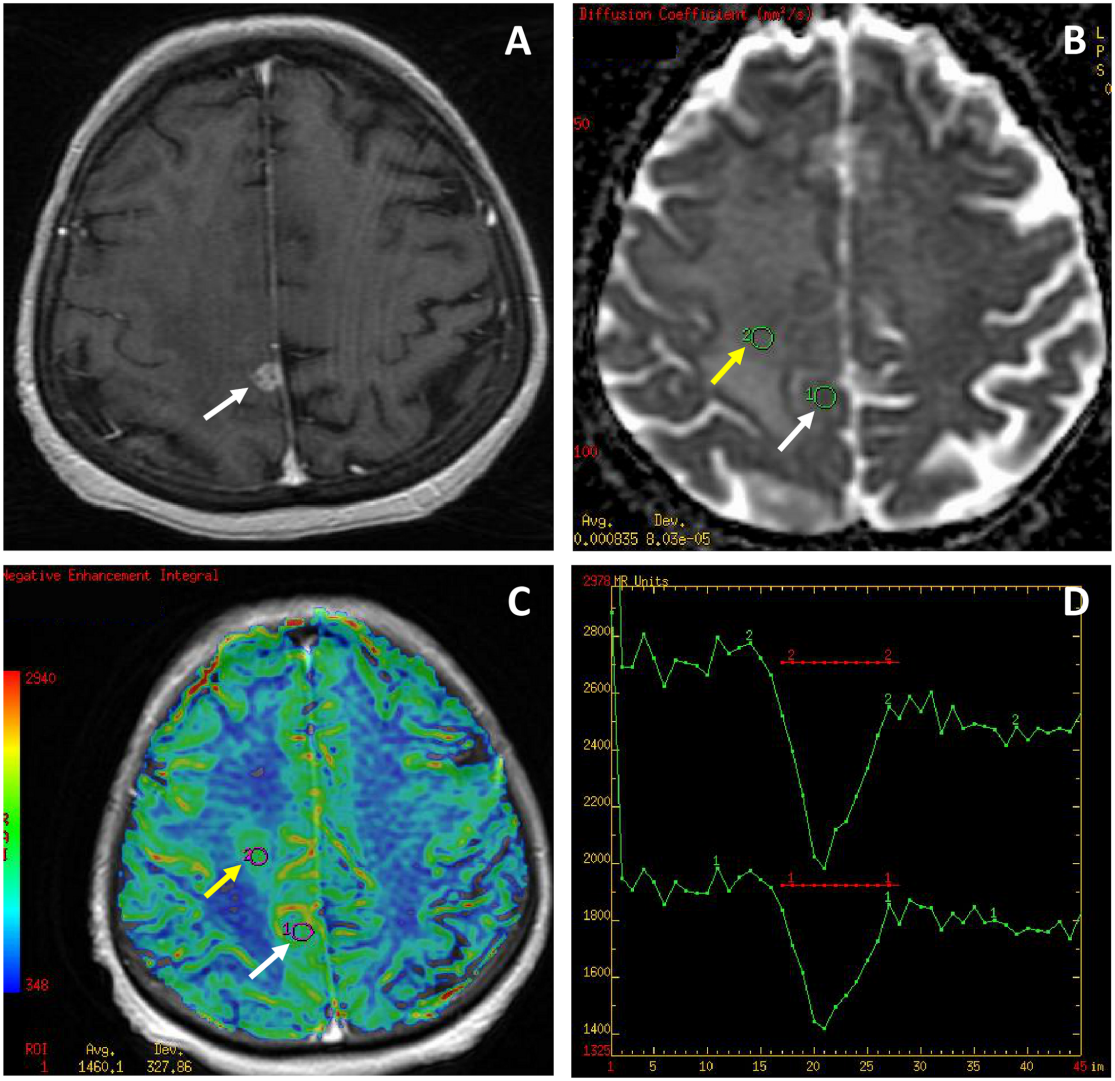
Glioblastoma multiforme (GBM). (A) a post-contrast T1-weighted image, (B) an ADC map, (C) a CBV map, (D) perfusion signal intensity time curves. The tumor appears as a small enhancing lesion (white arrows) with a large non-enhancing peritumoral zone mimicking a metastasis. The ADC map (B) shows minimal ADC values similar to normal white matter (0.83 x 10^−3^ mm^2^/s within the tumor core and 0.78 x 10^−3^ mm^2^/s within the peritumoral region of infiltration). The CBV map (C) shows the hyperperfused tumor core (white arrow, max rCBV = 2.9) and a large area of increased perfusion (yellow arrow, max rCBV = 2.25) within the peritumoral zone indicating neoplastic infiltration which is a feature differentiating GBM from a metastasis surrounded exclusively by a pure vasogenic edema. The perfusion curves (D) present only partial return to the baseline (red transverse line) in both the tumor core (lower curve) and the area of neoplastic infiltration (upper curve).
